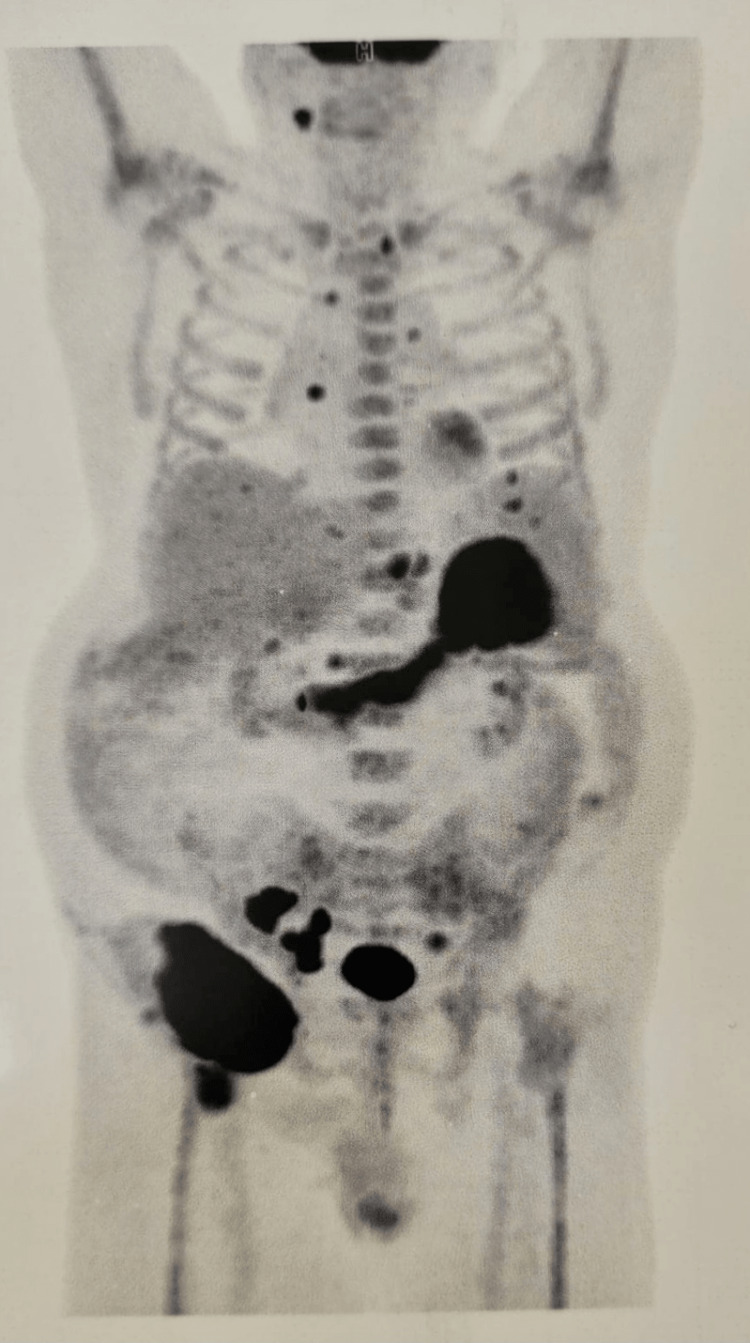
PET-CT result Intense uptake at the level of an inter gastrosplenic tumor mass (maximum standardized uptake value [SUVmax] = 16.13) goes further along the large curvature of the stomach and multiple uptaken lymph nodes situated above and under the diaphragm, isolated or organized as confluated masses, with more expressive masses located at the right inguinofemoral region (SUVmax = 26,08). There was also diffuse and inhomogeneous uptake at the level of the medulla throughout all scanned bones, suggesting myeloproliferative neoplasms (MPNs).
